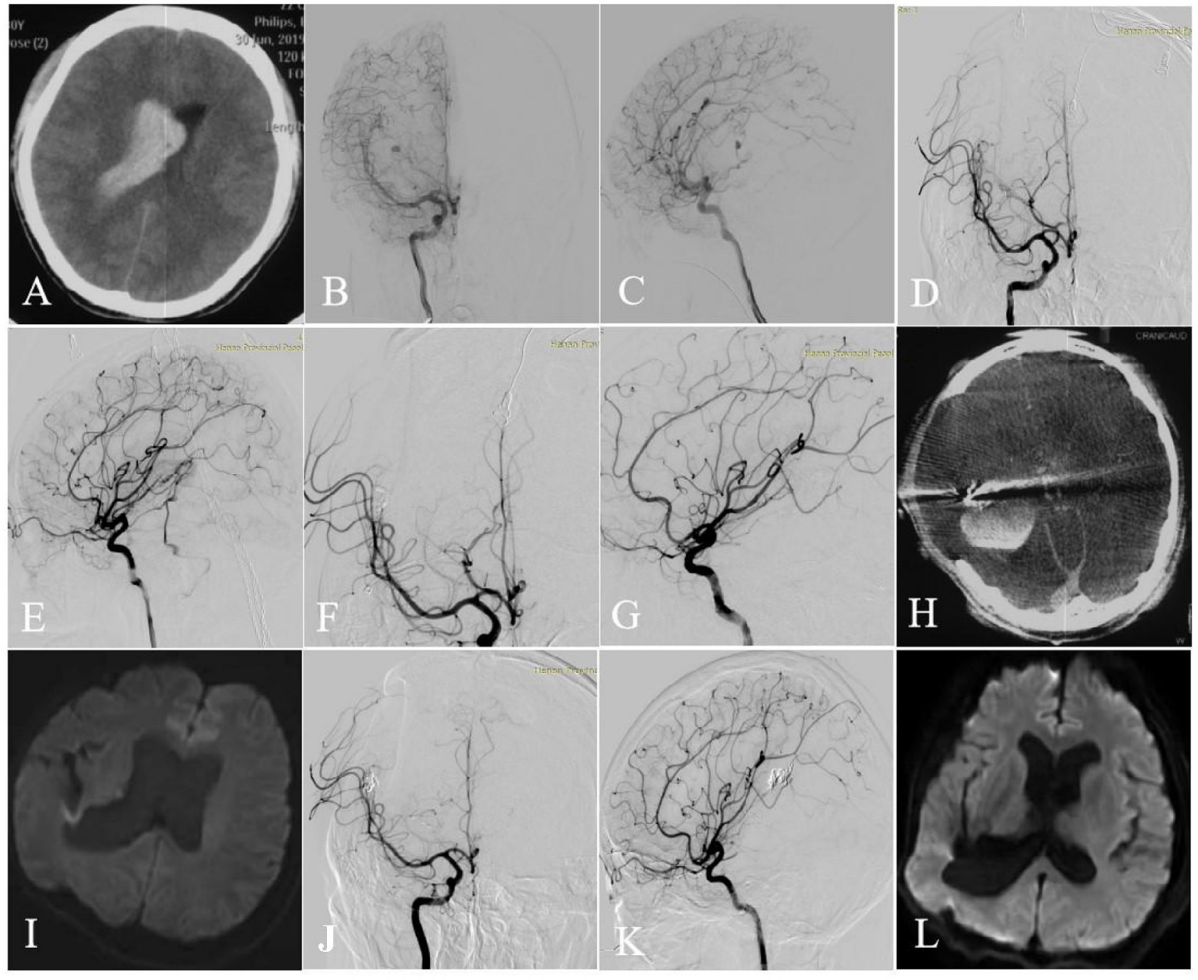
Axial CT image showed intraventricular hemorrhage (A). Selective DSA of the right ICA [anteroposterior (B) and lateral (C) views] showed that the AVM with an aneurysm (flow related pedicle) located at the temporal lobe was fed by the branches and perforators of ACA, MCA, and ICA and drained a single venous outlet via the cortical cerebral vein. After 3 months, TVE was performed. First, targeted embolization of the aneurysm [anteroposterior (D) and lateral (E) views]. And then the AVM was completely angiographically obliterated at the end of the operation [anteroposterior (F) and lateral (G) views]. Intraoperative intracerebral hemorrhage occurred (H, Dyna CT). No cerebral infarction was seen on Axial DWI performed 3 days (I) after surgery and then 6 months (L). The AVM was completely angiographically obliterated at the 6-month follow-up [anteroposterior (J) and lateral (K) views].
